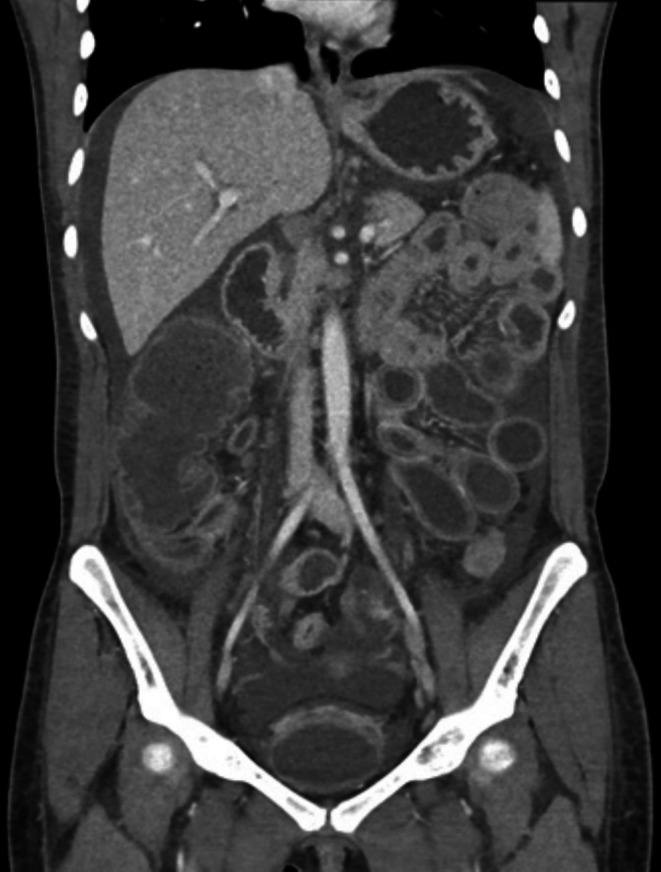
CT scan prior to therapy: bladder edema, pancolitis, and terminal ileitis with mucosal hyperenhancement of the bowel and moderate ascites.
